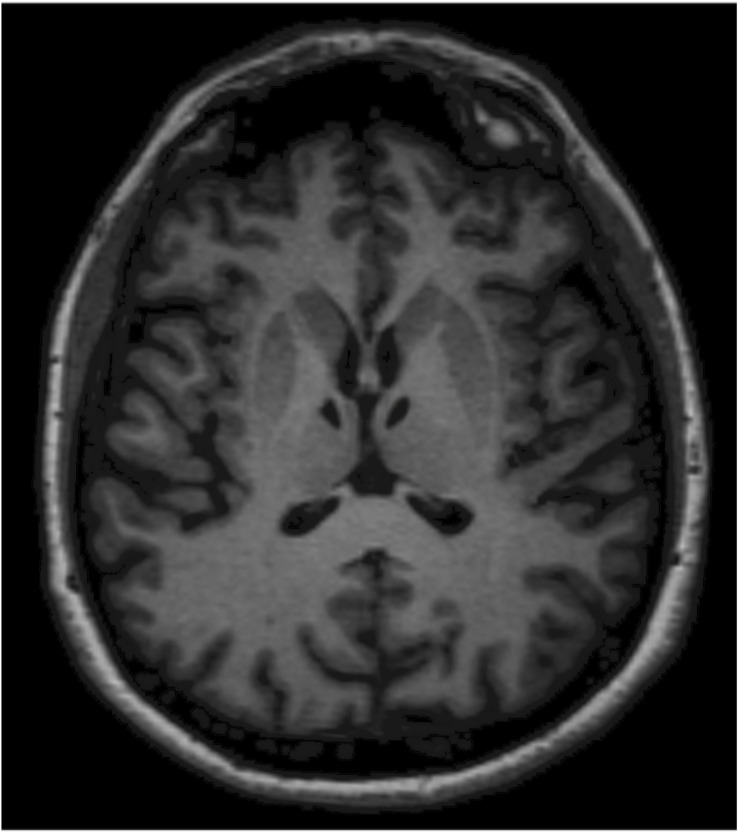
Magnetic resonance imaging (MRI) shows lacunar thalamic infarcts symmetrically located within the polar and paramedian vascular territories of both thalami. Lesions are well-demarcated and isointense to cerebrospinal fluid as observed in chronic settings. The anatomical symmetry was the distinctive feature in this patient.
